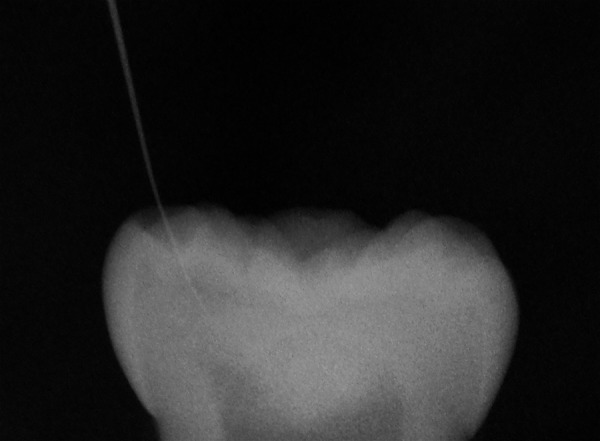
X-ray of tooth 85 after extraction. Note: The exterior opening of the hidden caries was located using a #8 file that entered from the mesial central fissure reaching the pulp horn
